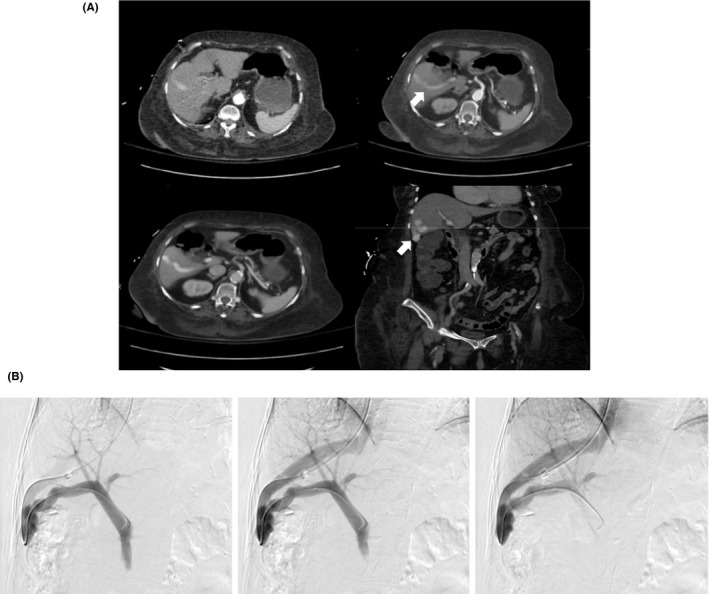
A 75‐year‐old woman with congenital intrahepatic portosystemic venous shunt. A, Pre‐embolization axial and coronal images from a contrast‐enhanced CT of the abdomen and pelvis which demonstrates an enlarged right posterior portal vein and right hepatic vein with significant enhancement in the arterial phase. There is vascular tortuosity at the level of the shunt (white arrow). B, Portal venography with fluoroscopic DSA obtained with transjugular access and catheter positioning across the intrahepatic portosystemic shunt into the main portal vein. This venogram confirmed the communication between the right hepatic and right posterior portal veins
